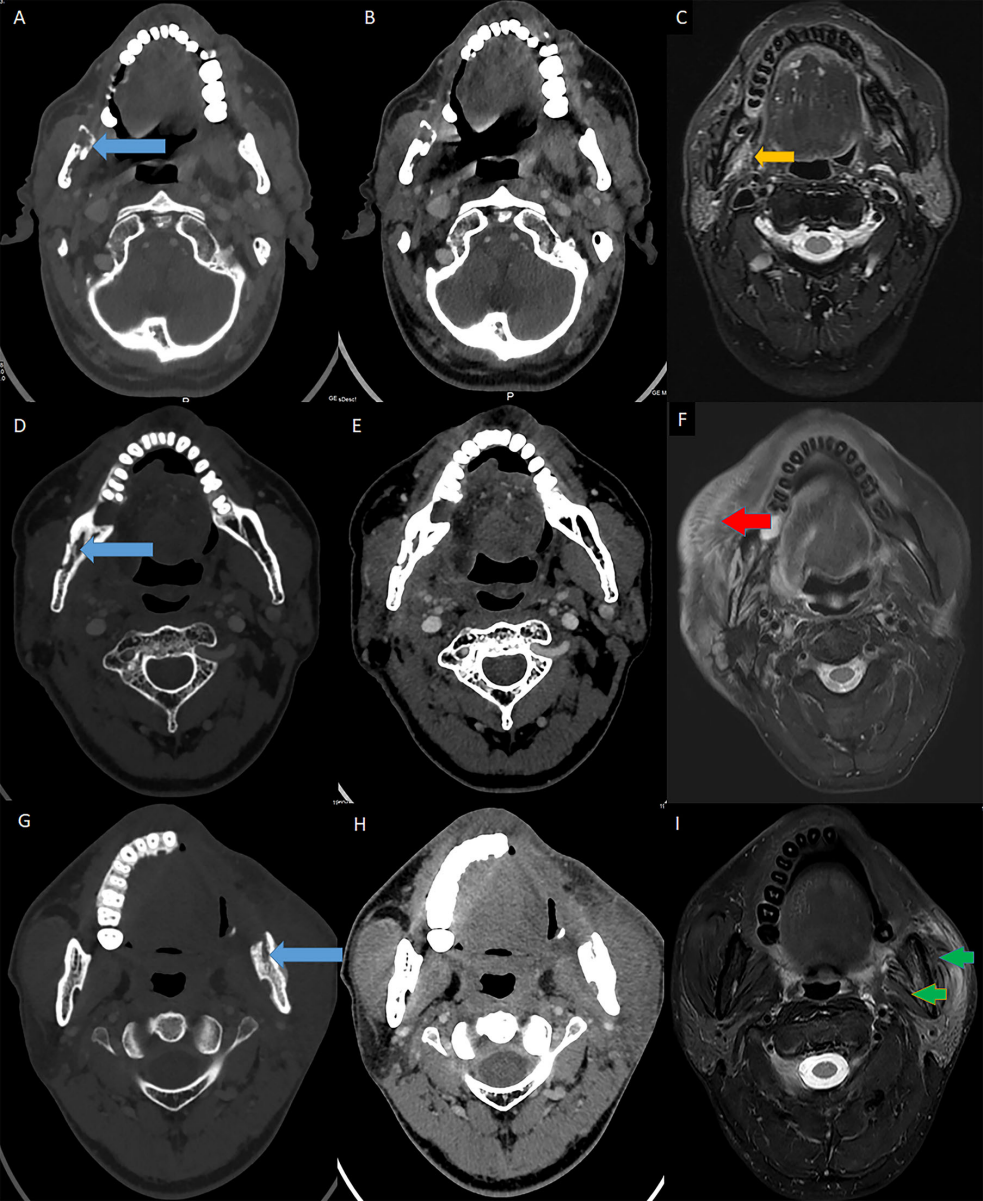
MRI evidence for muscular STI in ORN patients. Blue arrow: ORN lesions; Orange arrow: muscular hypertrophy; Red arrow: muscular edema; Green arrow: muscular atrophy. (A) The axial enhanced CT (bone window) showed the ORN lesion in the ramus. (B) The axial enhanced CT (soft-tissue window) revealed both the ORN and soft tissue content. (C) The axial T2-weighted MRI showed hypertrophy in the pterygoid muscles due to STI. (D) The axial enhanced CT (bone window) showed the ORN lesion in the body and ramus (the second patient). (E) The axial enhanced CT (soft-tissue window) revealed both the ORN and soft tissue content (the second patient). (F) The axial T2-weighted MRI showed edema in the ipsilateral masseter muscles due to STI (the second patient). (G) The axial enhanced CT (bone window) showed the ORN lesion in the ramus (the third patient). (H) The axial enhanced CT (soft-tissue window) revealed both the ORN and soft tissue content (the third patient). (I) The axial T2-weighted MRI showed atrophy in both the pterygoid and masseter muscles due to STI (the third patient).
